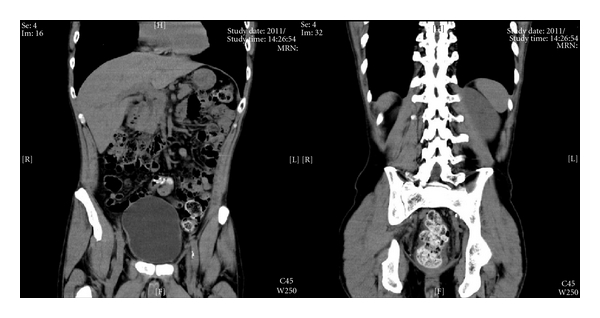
Coronal computed tomography scan showing the amount of colon gas and a large stool mass blocking the rectum.
